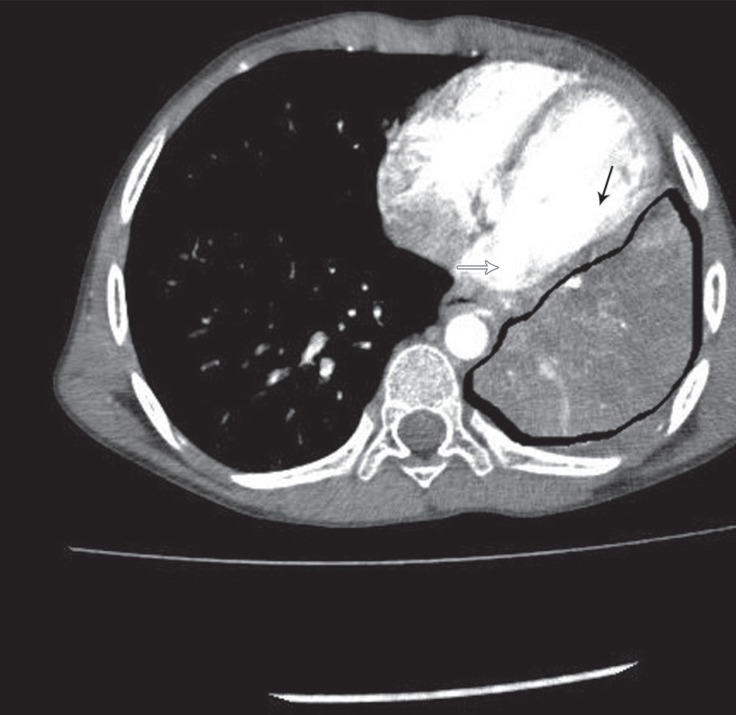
Computed tomography image of the inflammatory myofibroblastic tumor, invading the left atrium. White thick arrow: left atrial wall invaded by the tumor; black thin line: left ventricular wall; area surrounded by the black thick line: inflammatory myofibroblastic tumor with the lower lobe
